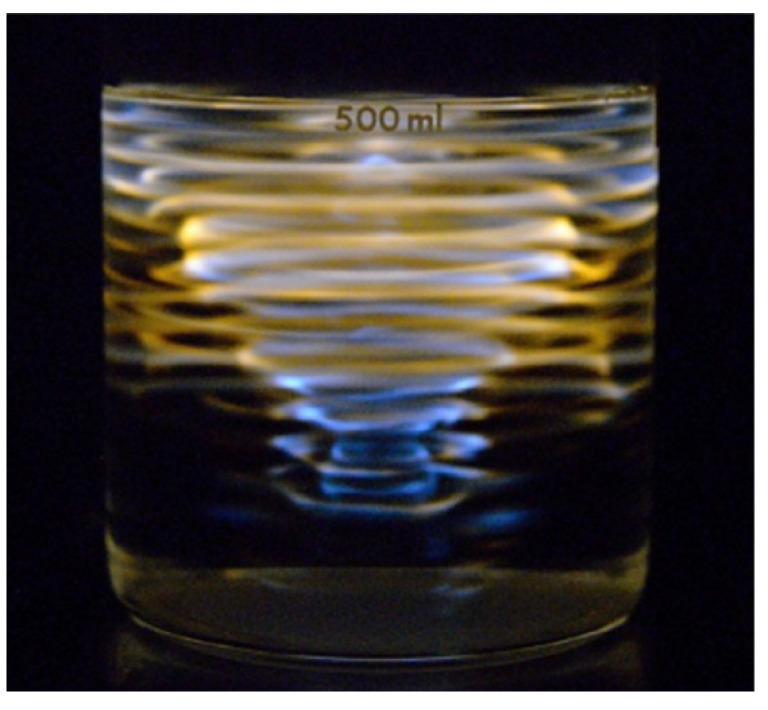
Photographic image of MBSL from Ar-saturated SDS solution at an acoustic power of 4 W [3]. Ultrasound at a frequency of 148 kHz was irradiated from the bottom of the beaker. Copyright 2015, with the permission from Elsevier.
